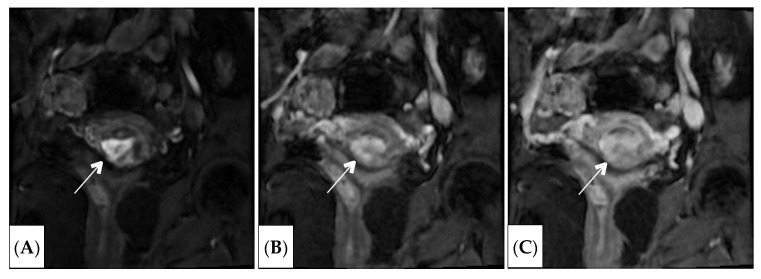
MRI T1WI FS with intravenous bolus contrast (Clariscan 0.2 mL/kg body weight) in the early arterial (A), parenchymal (B), and delayed (C) phases, revealing early hypervascular enhancement of the chorion (indicated by the arrow) and pronounced thinning of the underlying uterine wall.
